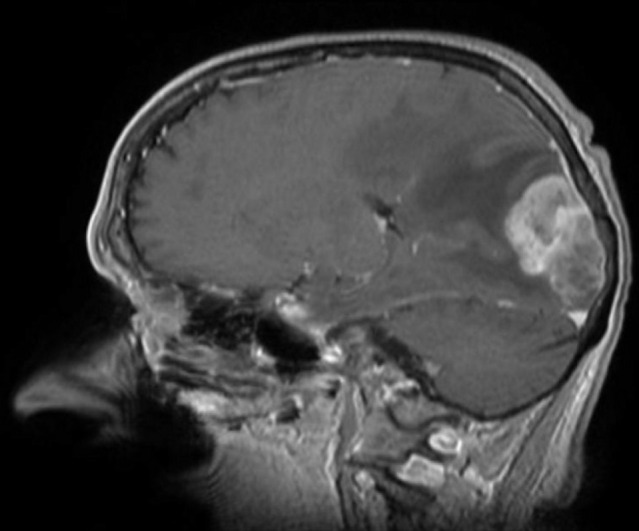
Gadolinium-enhanced T1-weighted MRI scan, sagittal section, showing a large left parieto-occipital extra-axial tumor, with a characteristic dural tail sign and peritumoral edema, suggestive of meningioma.
